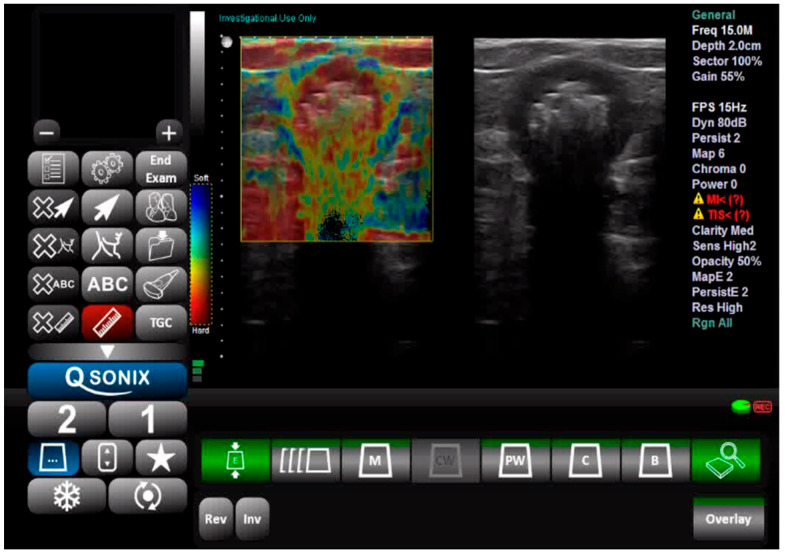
Ultrasound and elastography assessment of the sigmoid colon.
